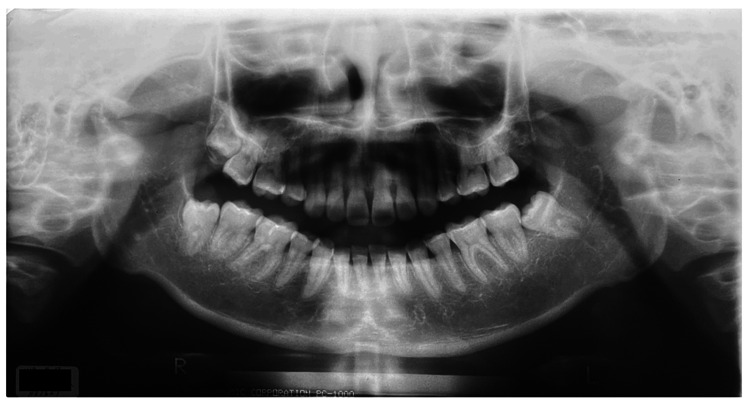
Panoramic radiography at the initiation of treatment in Case 1
